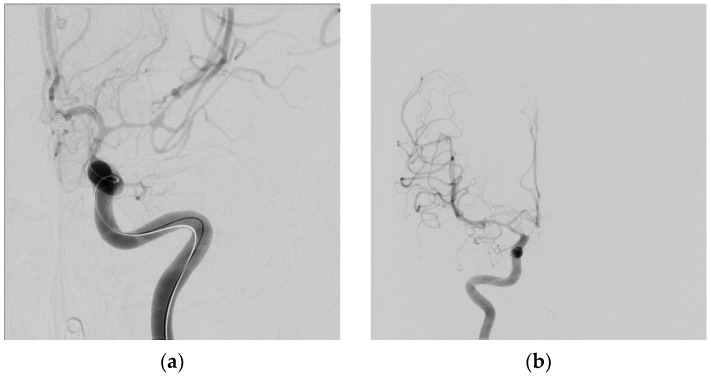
Seven days later, the patient became more lethargic with a new right-sided weakness. Velocities in the middle cerebral arteries on transcranial Doppler exceed 200 cm/s, indicating significant vasospasm. Hypertension was induced (see text), and after a CT ruled out cerebral infarction, a following catheter angiogram showed severe left MCA narrowing (a), where the thickest subarachnoid clot was located on the initial CT, and moderate vasospasm of the right MCA (b).
